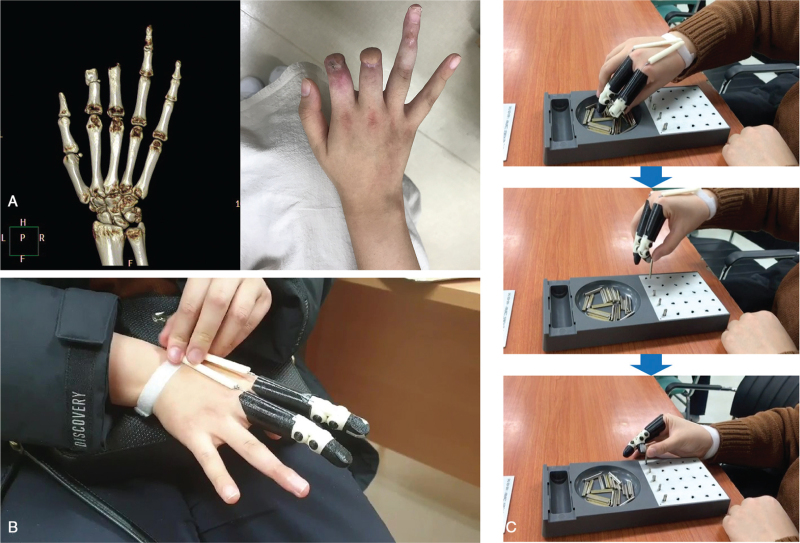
The case of patient 1. (A) A 3D-reconstructed bone structure from a computed tomographic scan and gross appearance of the right hand in patient 2. The amputation levels correspond to second and third proximal phalanges. (B) The patient is wearing the fabricated body-powered 3D-printed finger prostheses. (C) The patient is performing peg board training with the finger prostheses.
